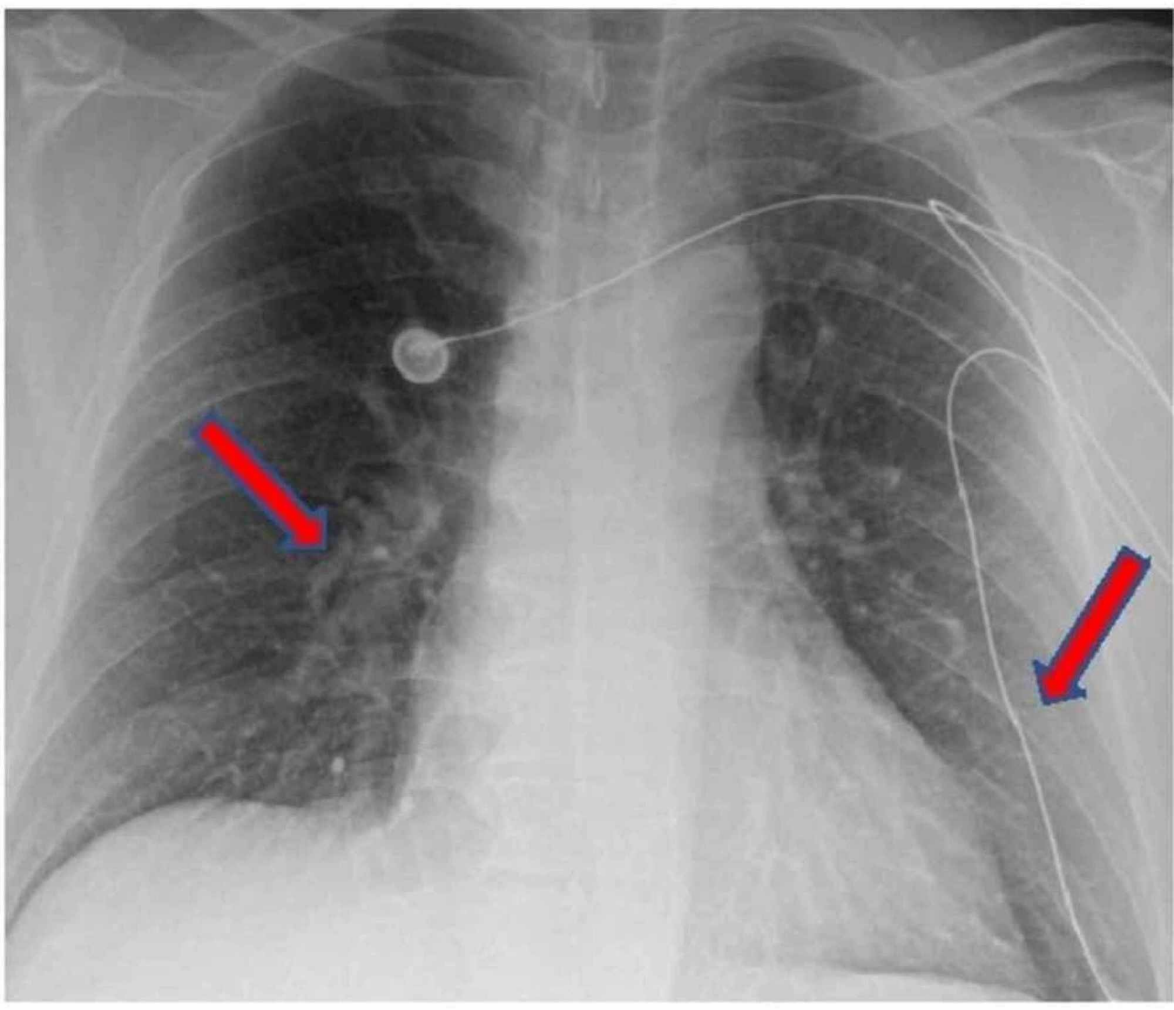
CXR of case 3 showing bilateral patchy infiltrates. CXR: Chest X-ray
